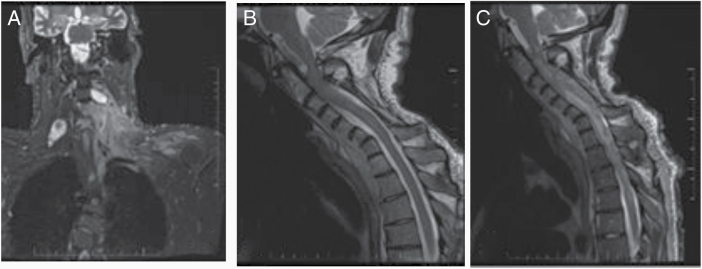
Imaging of Case 1 (A, B, C). (A) Case 1. MRI June 2018, shows neurofibroma in neck; (B) Case 1. MRI April 2019, normal cord; (C) Case 1. MRI June 2019 complete cord effacement and filling of the spinal canal with tumor.
